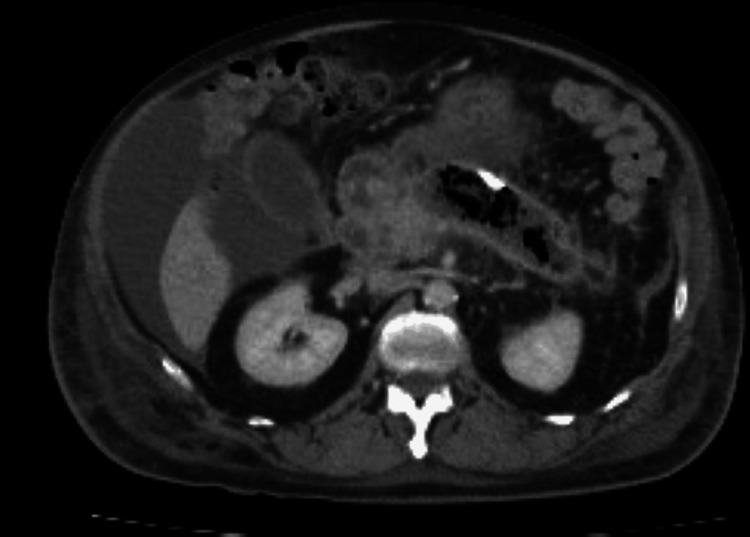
Axial CT of the abdomen showing persistent peripancreatic fluid collections
